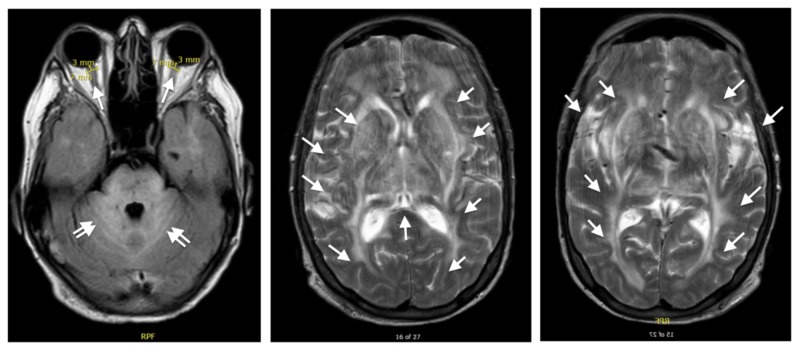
Brain MRI Fluid-attenuated inversion recovery (FLAIR) MRI showed hyperintense cerebellar white matter and brainstem changes (left image, double arrows). Measurement of optic nerve sheath diameter (ONSD) on MRI confirmed bedside ultrasonography findings of >6.5 mm ONSD bilaterally (left image, single arrows). Extensive diffuse bilateral white matter hyperintensity throughout the parietal, occipital, and temporal lobes seen on T2-weight MRI (middle and right image, arrows).
